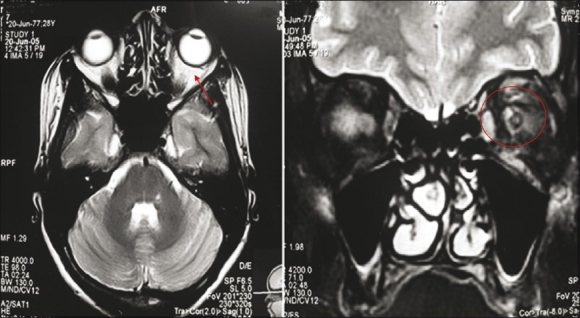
MRI: edema in the left extraocular muscle cone and retrobulbar fat extending into the orbital apex and subtle hyperintensity of the left optic nerve in the intraorbital and intracanalicular portion
